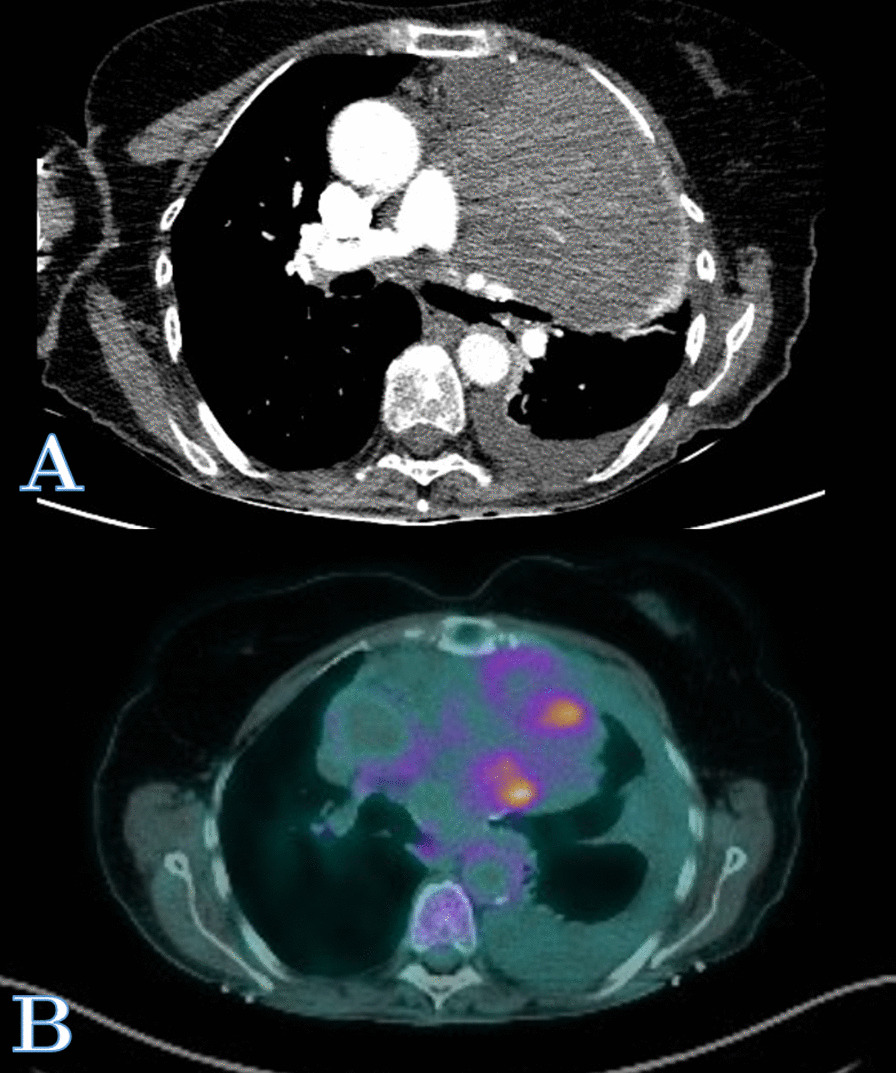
A Computed tomography scan with intravenous contrast revealing large bulky tumor with associated left pleural effusion present in the left hemithorax. B Positron Emission Tomography scan showing moderate hypermetabolism of the SFTP
